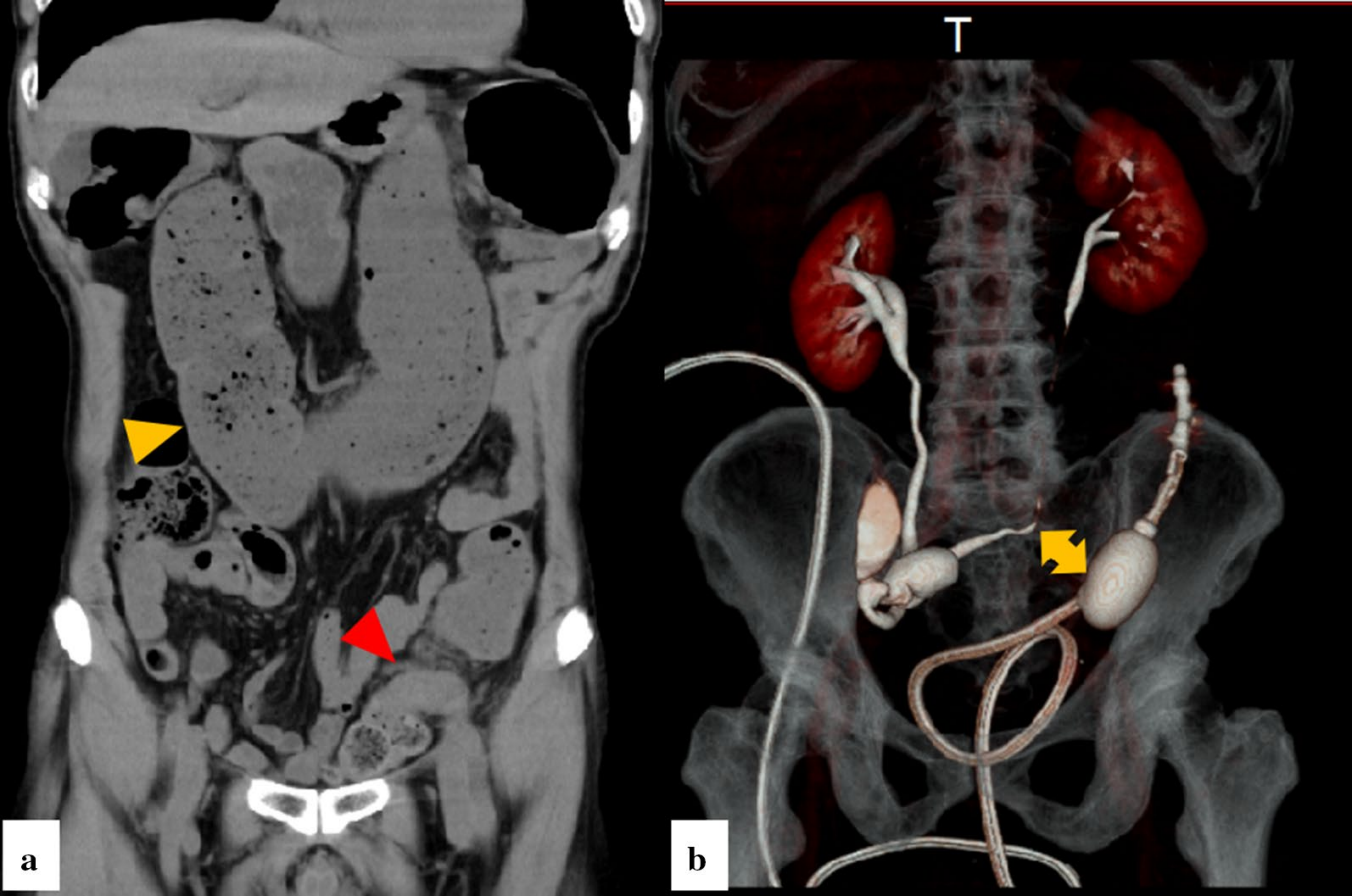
Preoperative computed tomography. a Computed tomography (CT) confirms colon dilation with fecal impaction from the ascending colon to the sigmoid colon (yellow arrowhead) and wall thickening in the sigmoid colon (red arrowhead). b CT colonography demonstrates that the left ureter was near the sigmoid tumor (yellow arrow)
